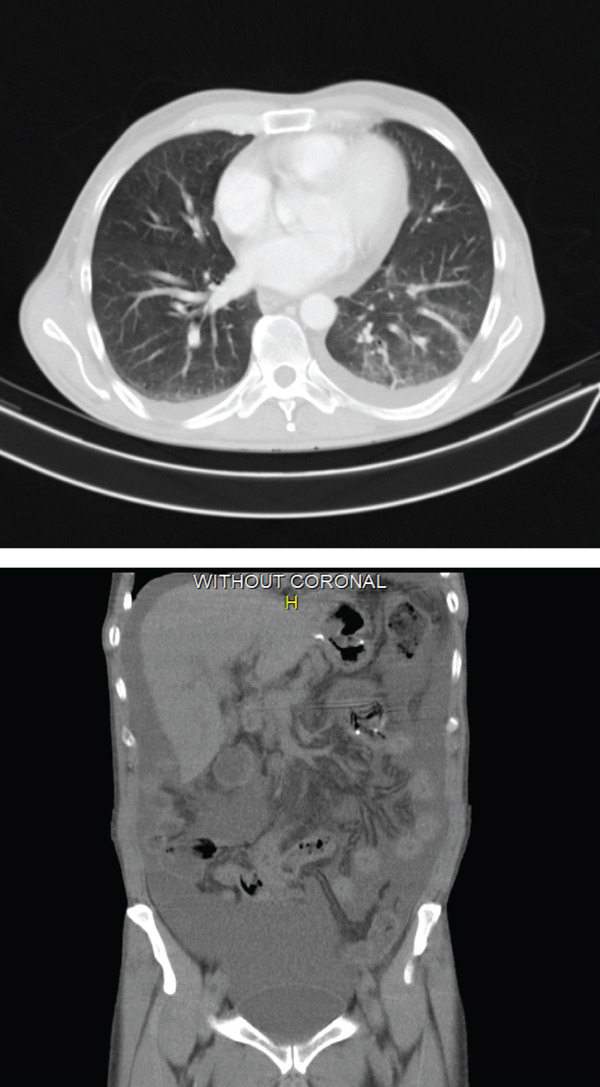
CT during ascites showing pleural effusion and free fluid.
